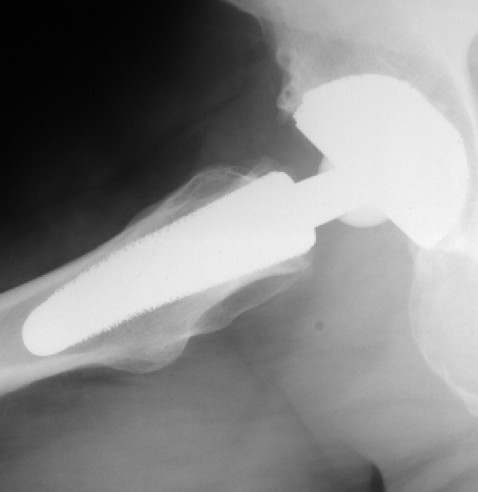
sagital radiograph of the Cut prosthesis. Figure 2 is showing the anteroposterior, figure 3 is showing the sagital radiograph 12 months after implantation of the implant in a 50 year old male patient.
